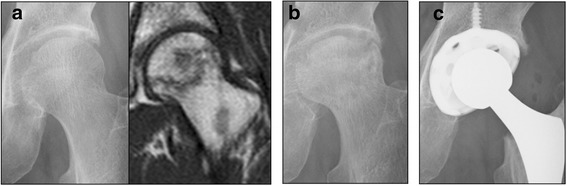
Case 1. Sixty-seven years old man with NOFH. a Anteroposterior radiograph and magnetic resonance imaging (T1WI) at the first examination showing NOFH. After diagnosis, alendronate was administered. b Collapse of the femoral head has progressed. c Eventually, THR was performed
